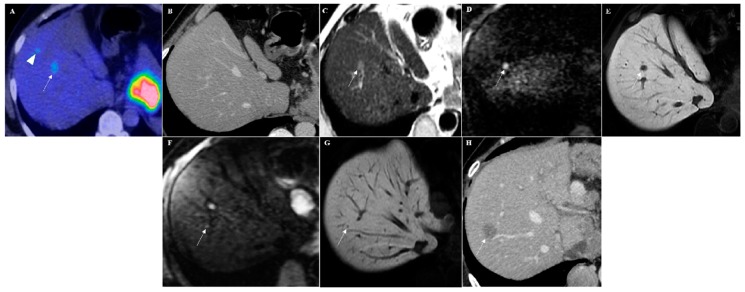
A 61-year-old male with a clinical history of gastric cancer. (A) 18-fluorideoxyglucose positron emission tomography (^18^FDG-PET) image shows two focal lesions in the segment VIII of the liver with increased uptake (SUVmax 4.7) (arrowhead and arrow). (B) In the axial computed tomography (CT) scan image, no suspicious lesions were visible. Diffusion-weighted imaging (DWI)-Gd-EOB-DTPA magnetic resonance imaging (MRI) examination showed only one slight hyperintense focal lesion on T2-weighted images (arrow in (C)) with strong hyperintensity in diffusion-weighted image (arrow in (D)) and hypointensity during the hepatobiliary phase (arrow in (E)), suspected for a liver metastasis. Moreover, a very small (2 mm) focal lesion with hyperintensity on diffusion-weighted image (arrow in (F)) and hypointensity during the hepatobiliary phase (arrow in (G)) was also detectable cranially in the same liver segment. An axial contrast-enhanced CT scan performed after six months confirmed this hepatic metastasis (arrow in (H)).
